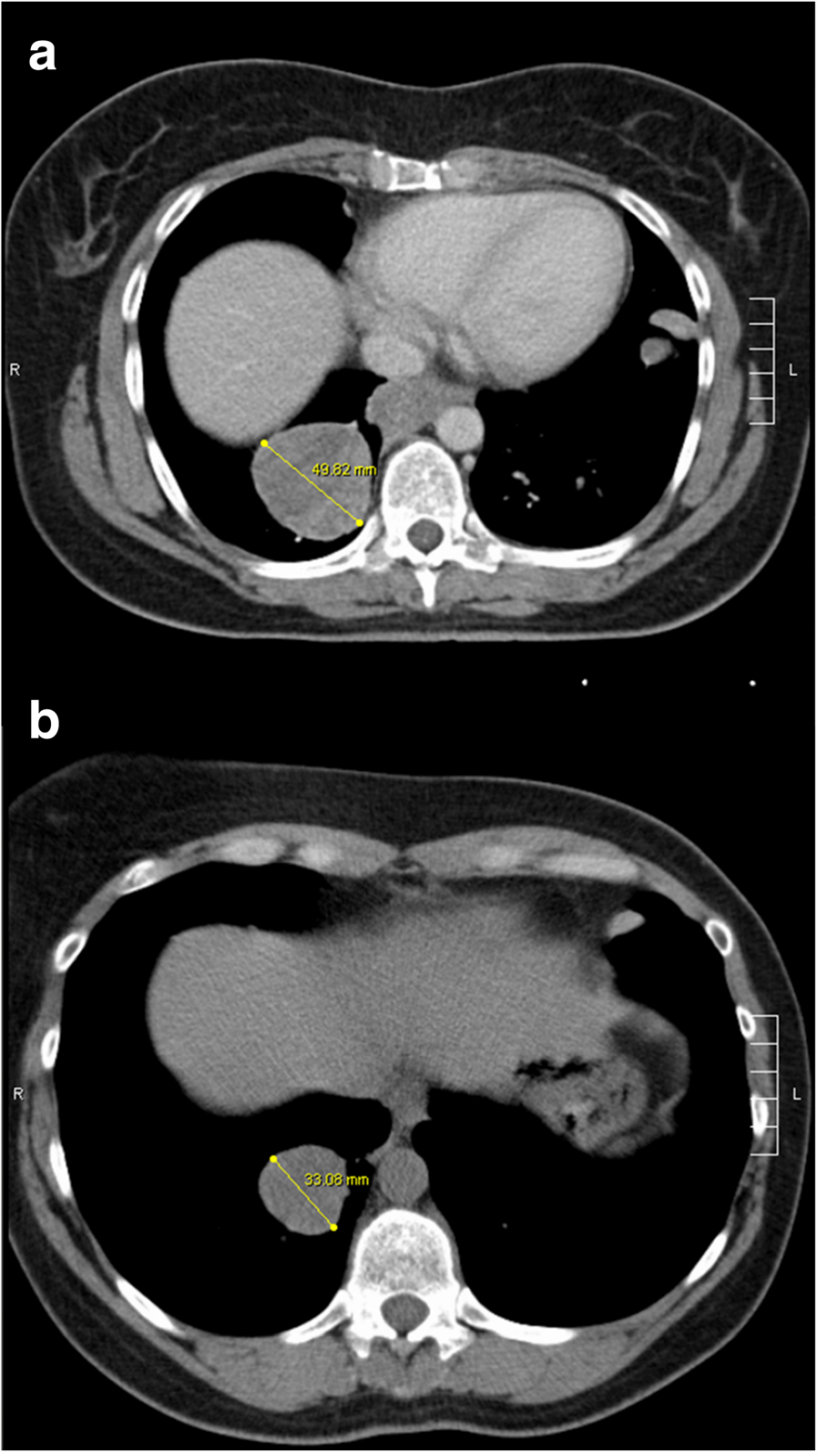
CT scans. Evaluation CT scans before start of pazopanib (a) and 12 weeks after start of pazopanib (so 6 weeks on pazopanib and 6 weeks off because of liver toxicity) (b) showing a shrinking pulmonary metastasis measured by largest diameter at both times
